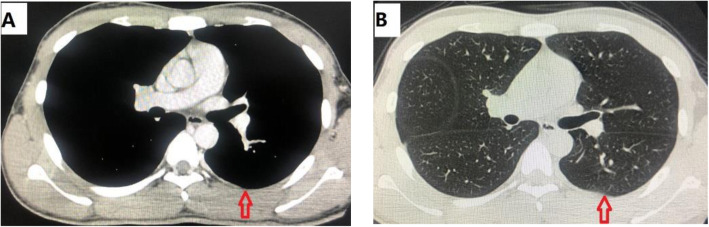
(A) 6 months after surgery; (B) 12 months after surgery. The patient followed up for the second and fourth time and chest CT showed no evidences of recurrence or lung metastases
